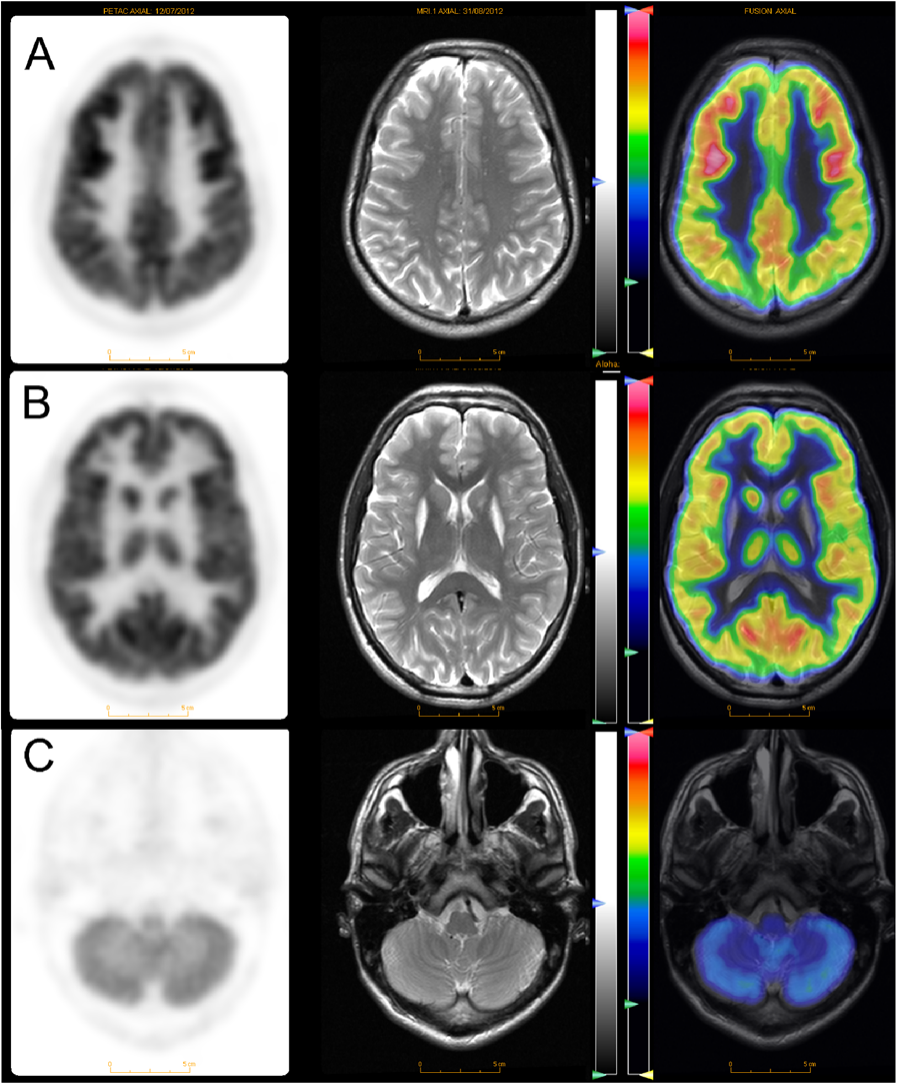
FDG-PET (left), axial T2 MRI (middle) and superimposed PET-MRI images of the brain showing frontal hypermetabolism (A), no detectable uptake in the putamina and reduced uptake in the left caudate (B) and hypometabolism in the cerebellum (C).
